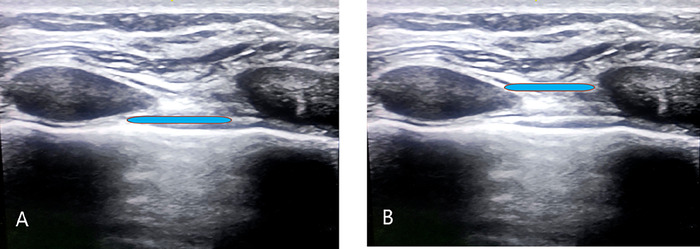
Ultrasound-guided local anesthetic placement for the (A) transversus thoracic muscle plane block and the (B) pecto-intercostal fascial block.
